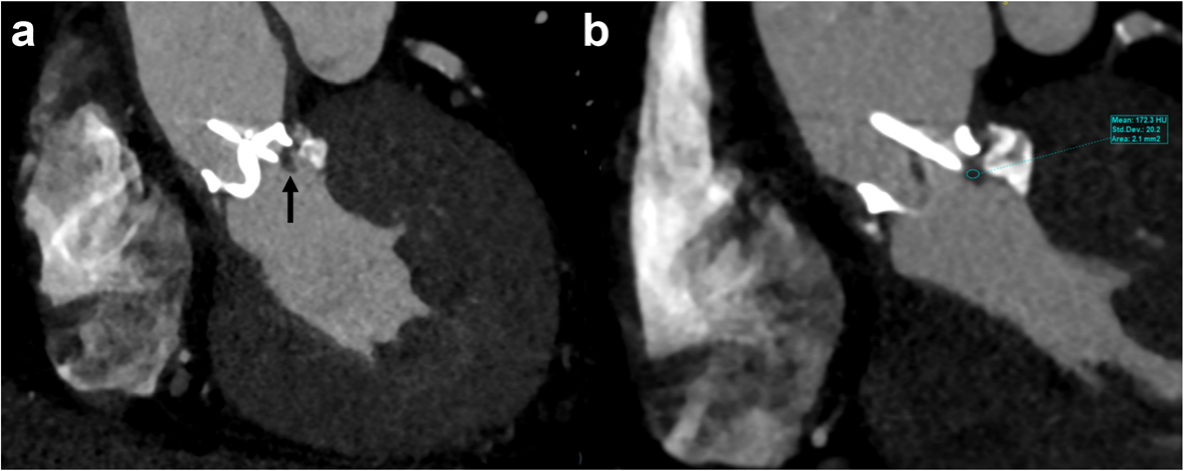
A 64-year-old male status post aortic valve replacement using Hall tilting disc valve (Medtronic, Inc., Minneapolis, Minn) and shortness of breath with increasing prosthetic aortic valve gradient on echocardiogram. Coronal oblique CTA reconstructed images reveal ovoid hypoattenuating lesion (Hounsfield unit, 172) at the inferior surface of the valve ring causing restricted valve opening, consistent with pannus formation
